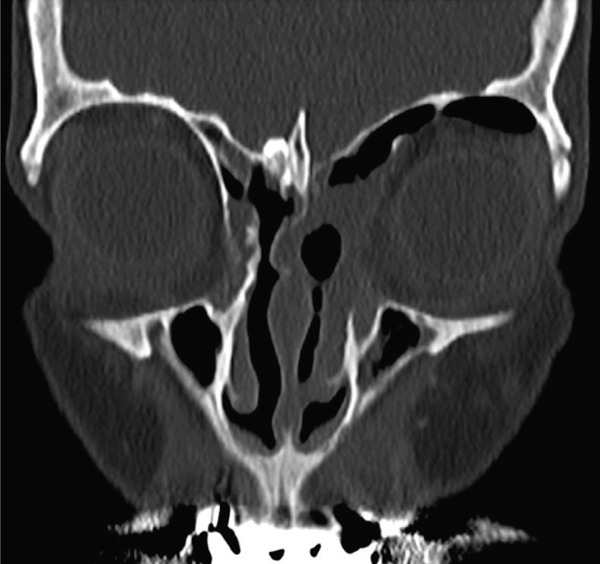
One‐year postoperative paranasal and orbital CT scan. Coronal projection: continuous opening of the medial meatus and ethmoidal sinus, along with pneumatization of frontoethmoidal mucoceles. The globe is in normal position.
